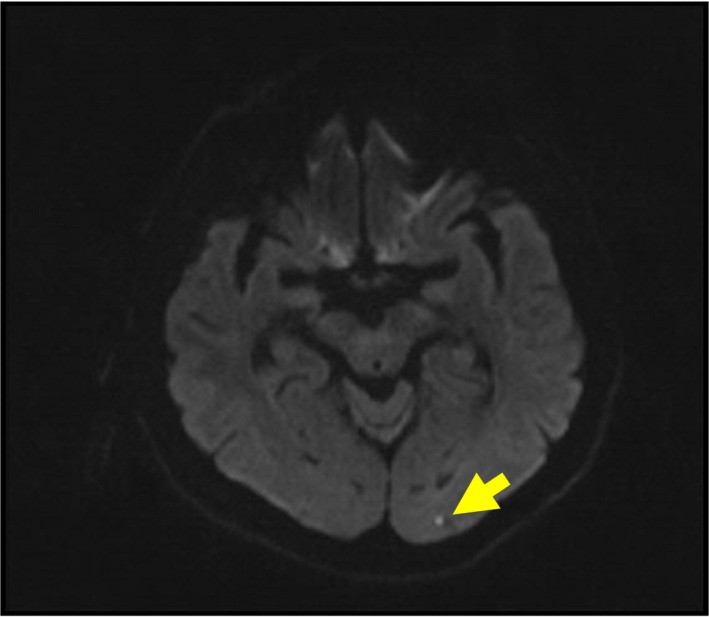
DWI left occipital lacunar infarct.
